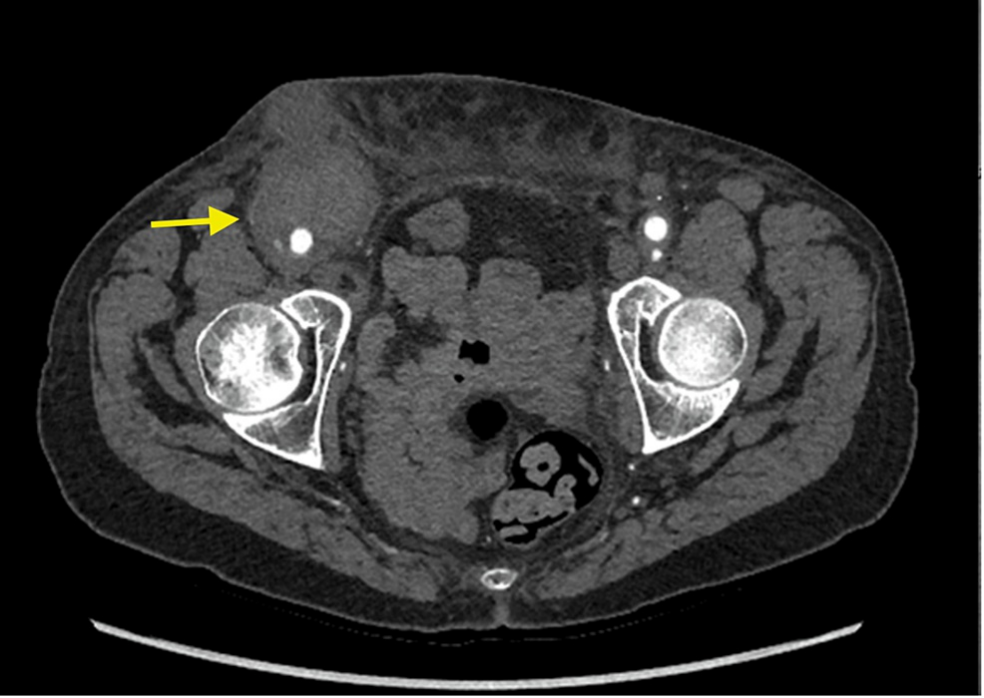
CT angiogram demonstrating a right femoral false aneurysm with surrounding suppuration
